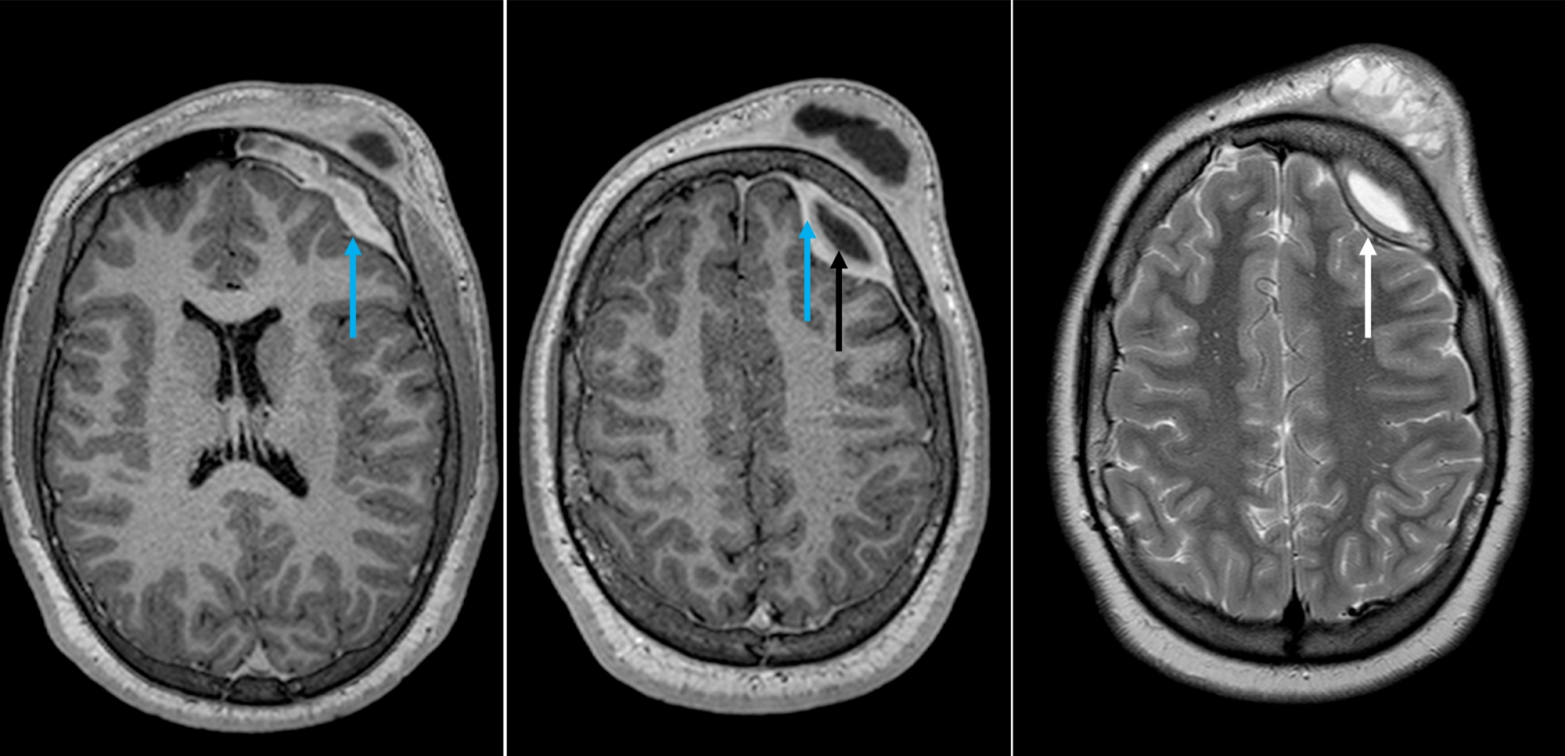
Preoperative MRI of the head: axial reconstruction Preoperative gadolinium-enhanced MRI of the head. Left and middle: An epidural contrast-enhanced capsule (blue arrow) with a center with low signal on a T1-weighted sequence was seen (black arrow), compatible with an abscess. Right: Note the dura mater (white arrow) interposed between the left frontal lobe and the epidural mass with a hyperintense signal on T2-weighted sequence. MRI: magnetic resonance imaging
